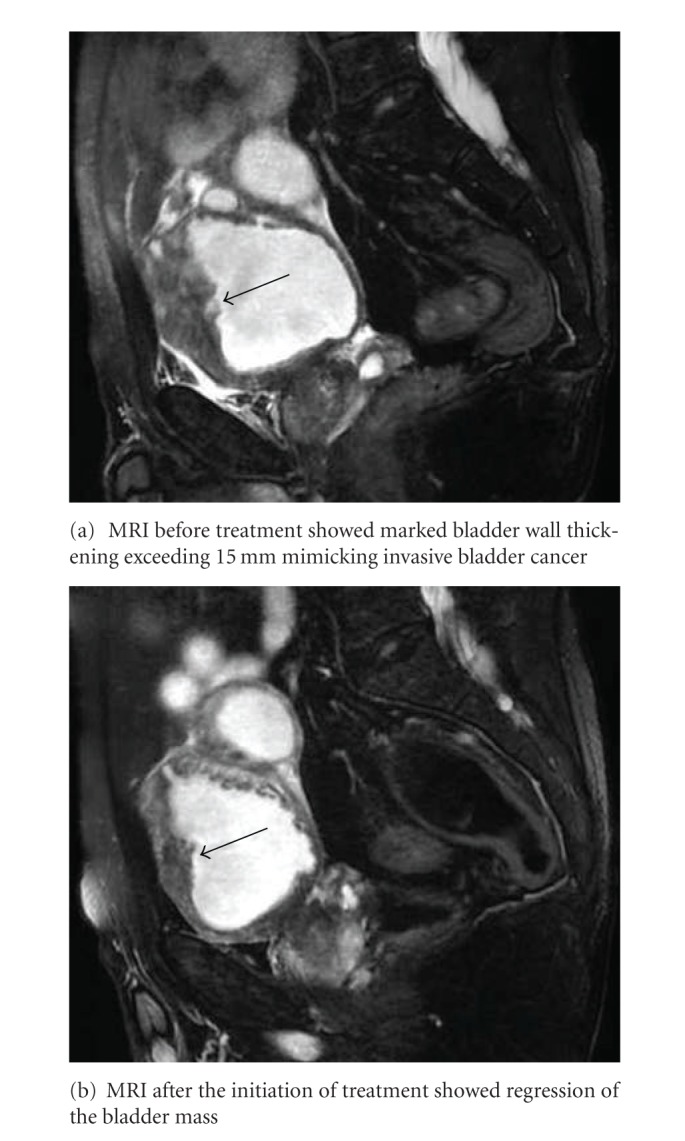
MRI findings (sagittal section). Arrow; bladder wall thickening.
